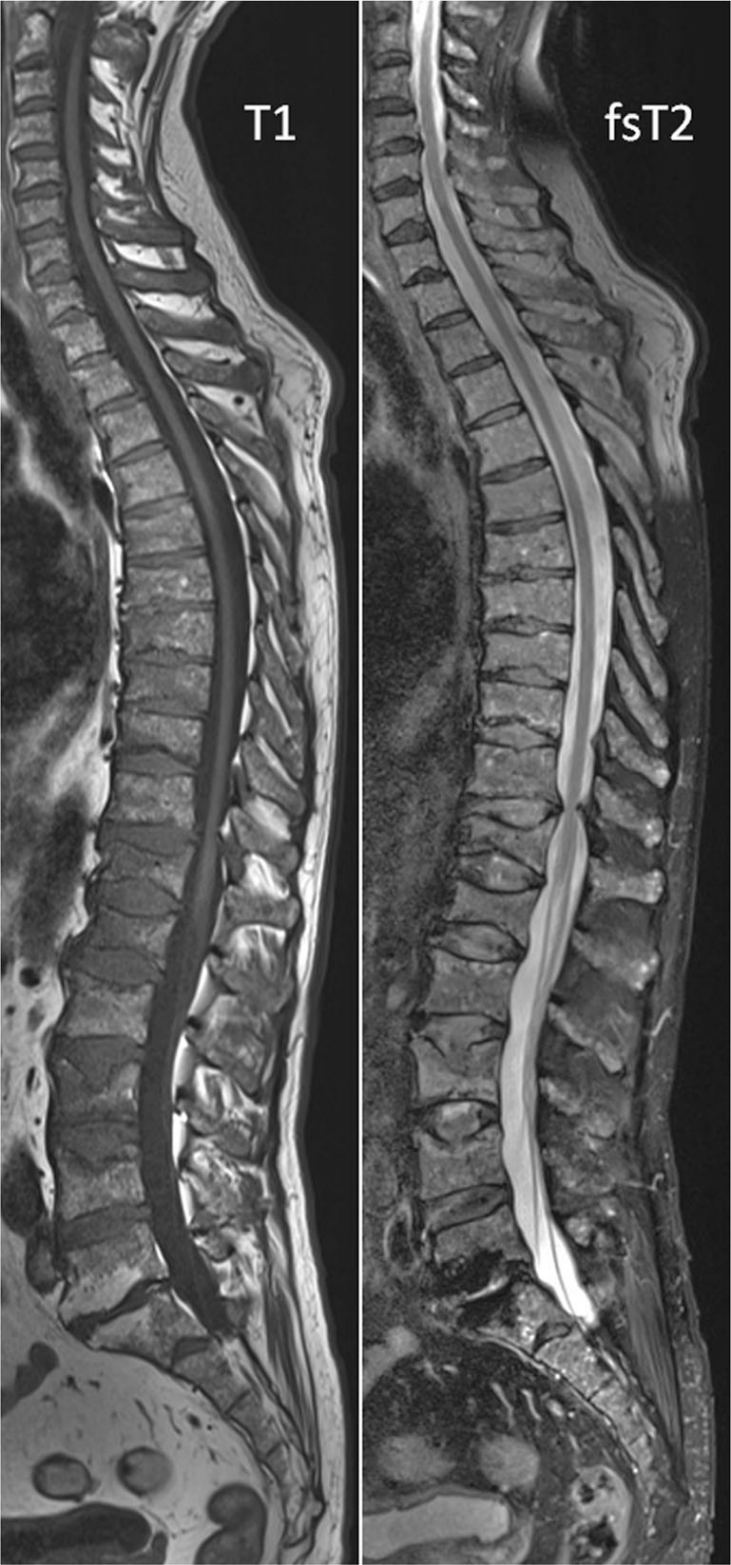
Sagittal T1-weighted (*left*) and fat-suppressed T2-weighted (*right*) images of the spine displaying a diffuse bone marrow infiltration of the cervical, thoracic, lumbar and sacral spine with low signal intensity on T1- and intermediate to high signal intensity on fat-suppressed T2-weighted images
